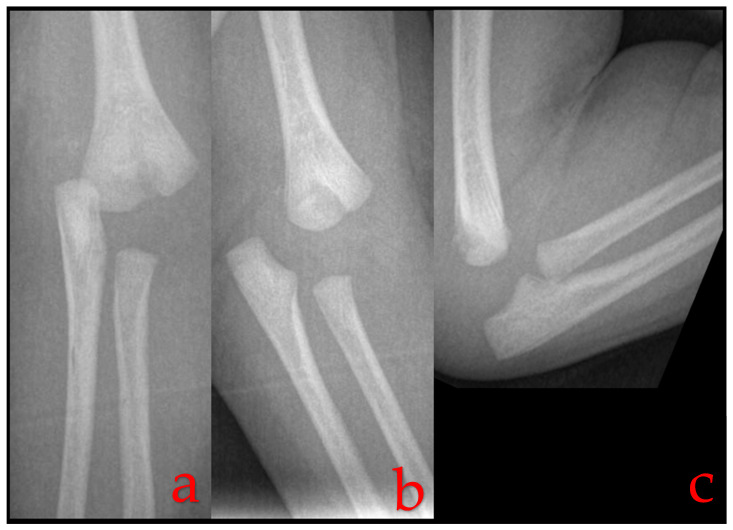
AP, (a) oblique (b) and lateral (c) elbow radiographs of a one-day-old male newborn with an injury attributed to the process of childbirth, revealing a misalignment between the distal humerus and the forearm—a characteristic indication of TPHS.
